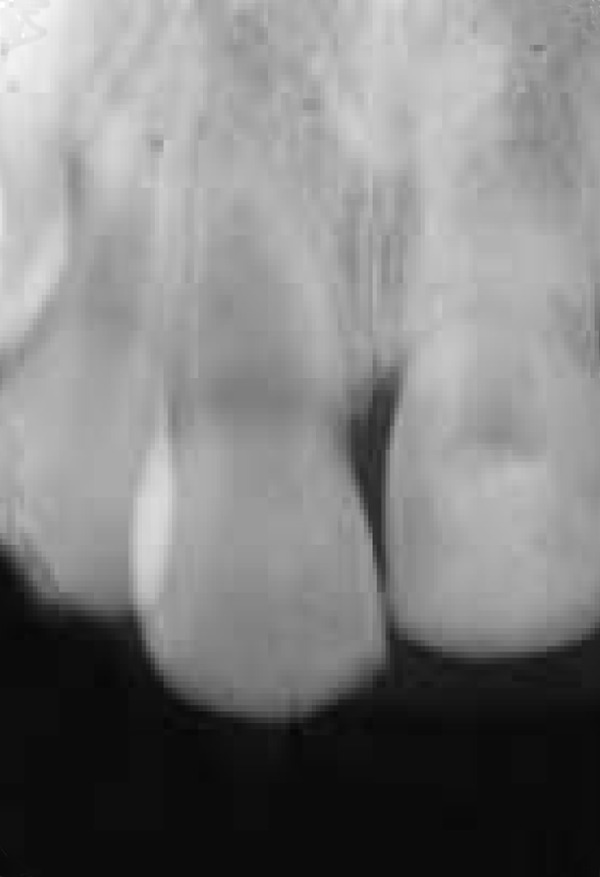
Follow-up radiograph taken 4 years after avulsion
